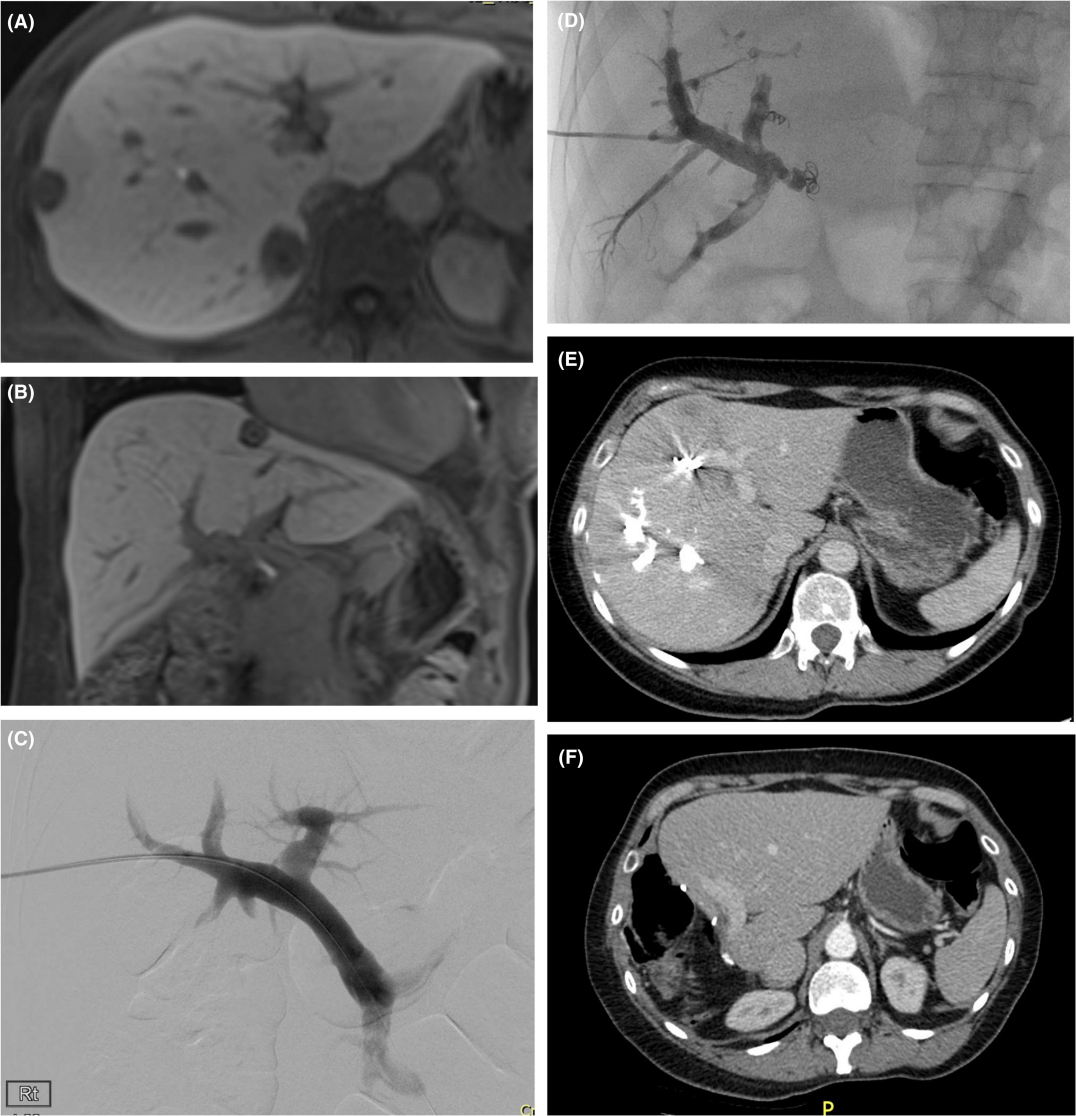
MRI showing multiple CRLM (A,B) requiring right hepatic trisectionectomy (resection of segments 4–8). PVE (C,D) resulted in FLR hypertrophy (E), allowing successful resection and further hypertrophy (F).
